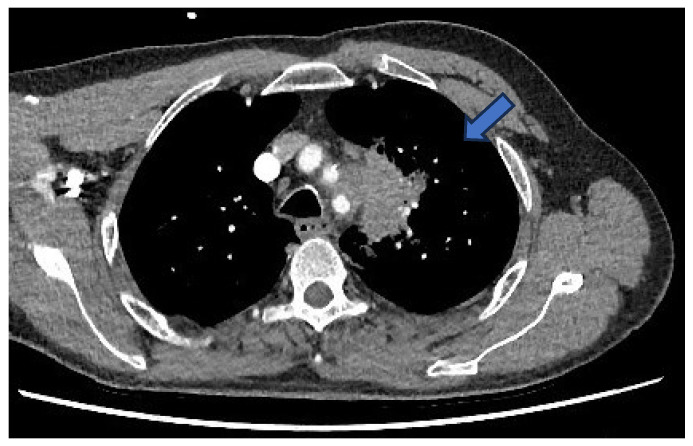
CT—left-sided lung mass (blue arrow) invading into the mediastinum, involving the aortic arch and proximal descending aorta.
